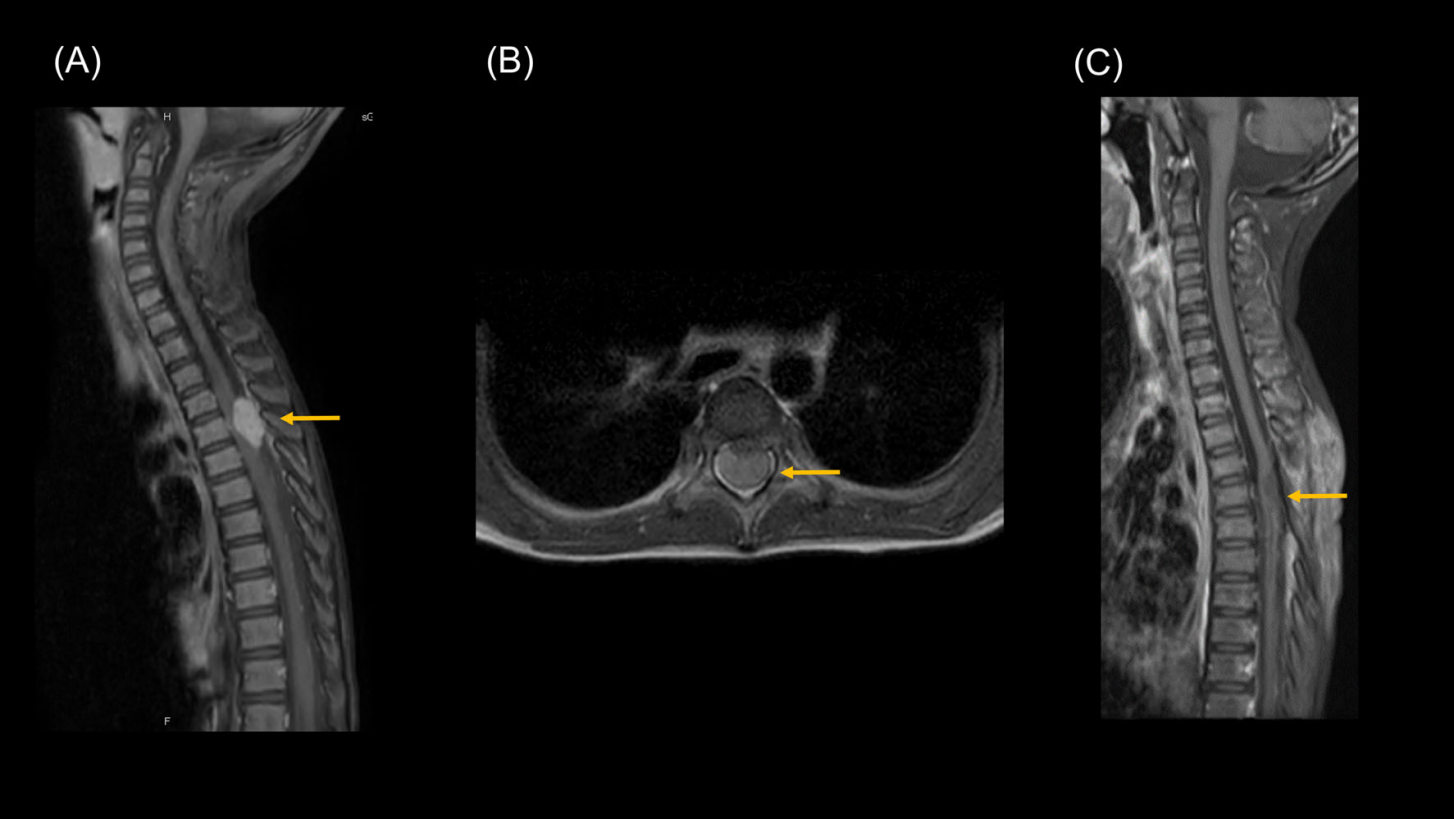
Gadolinium‐enhanced T1 weighted (Gd‐T1w) magnetic resonance imaging (MRI) at diagnosis. (A) The sagittal section revealed a mass with contrast enhancement at the T5 level. (B) The mass appeared relatively distinct from the spinal cord in the axial section. (C) Post‐removal, post‐radiochemotherapy, and pre‐high‐dose chemotherapy images show a good response to treatment.
